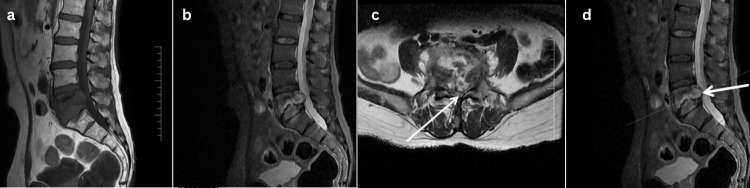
Spinal case 11 (a, b) MRI of the lumbar spine in the axial view and T2 sagittal views, respectively, showing severe T12-L1 disc retropulsion with compression of the conus medullaris and nerve roots, along with reactive inflammatory changes at this level. (c, d) Arrow mark indicates small epidural collection in the posterior aspect of the L5 vertebral body noted causing moderate canal stenosis. Data shown are representatives MRI findings from a single patient case (spinal case 11). Original images obtained by the authors at Subbaiah Institute of Medical Sciences, Shivamogga.
